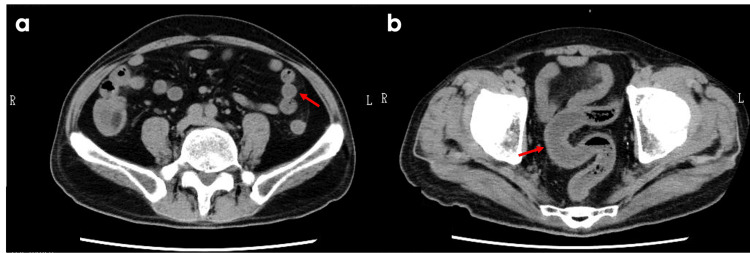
Computed tomography (CT) images after medical treatment (a) Axial CT image showing bowel wall edema localized in the small intestine (highlighted by red arrows), with no evidence of gastrointestinal perforation. (b) Axial CT image demonstrating continuous edema extending to the colon and rectum (arrows). Arrows indicate areas of bowel wall thickening and edema in both the small and large intestines.
